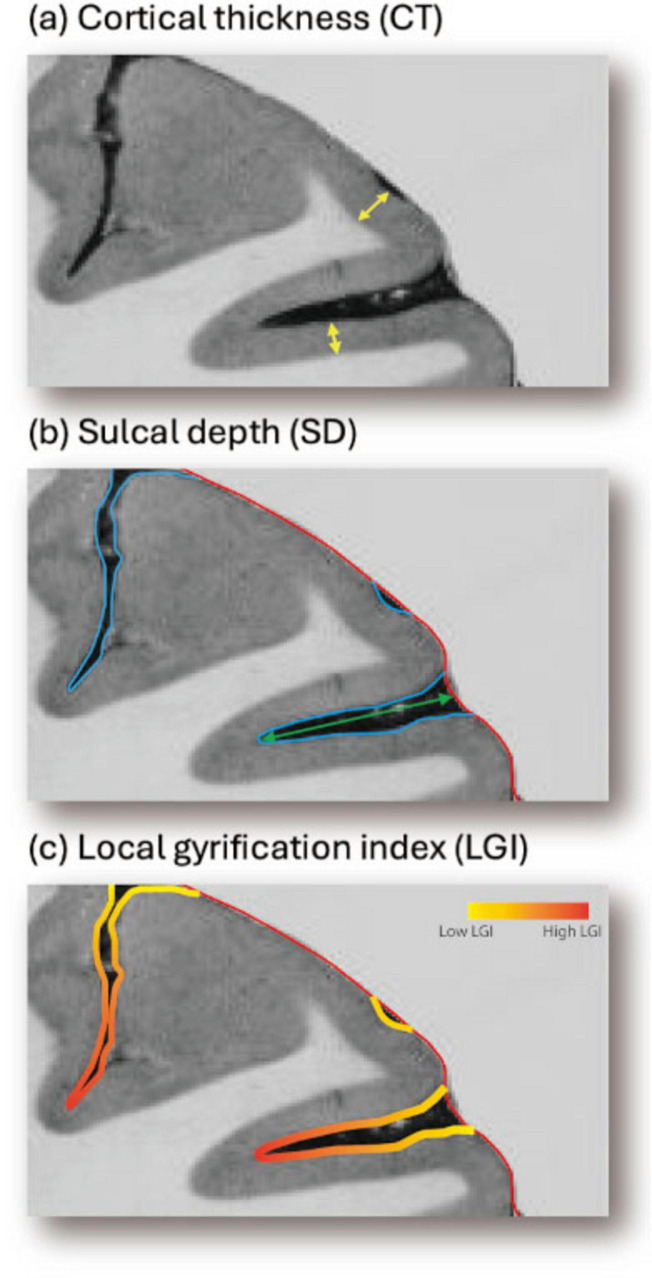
Overview of the methodology for measuring (a) CT, (b) SD, and (c) LGI on the cerebral surface. CT is measured as the minimal distance between corresponding point on the pial and white matter surface (yellow bar). SD is measured as the Laplacian trajectory between CHS (red contour) and the pial surface (blue contour). LGI is then measured as the ratio between the pial (blue) and CHS (red) surface area. The color gradients for LGI indicates the regions expected to have higher values (shown in orange) and lower values (shown in yellow). CHS, cerebral hull surface; CT, cortical thickness; LGI, local gyrification index; SD, sulcal depth
